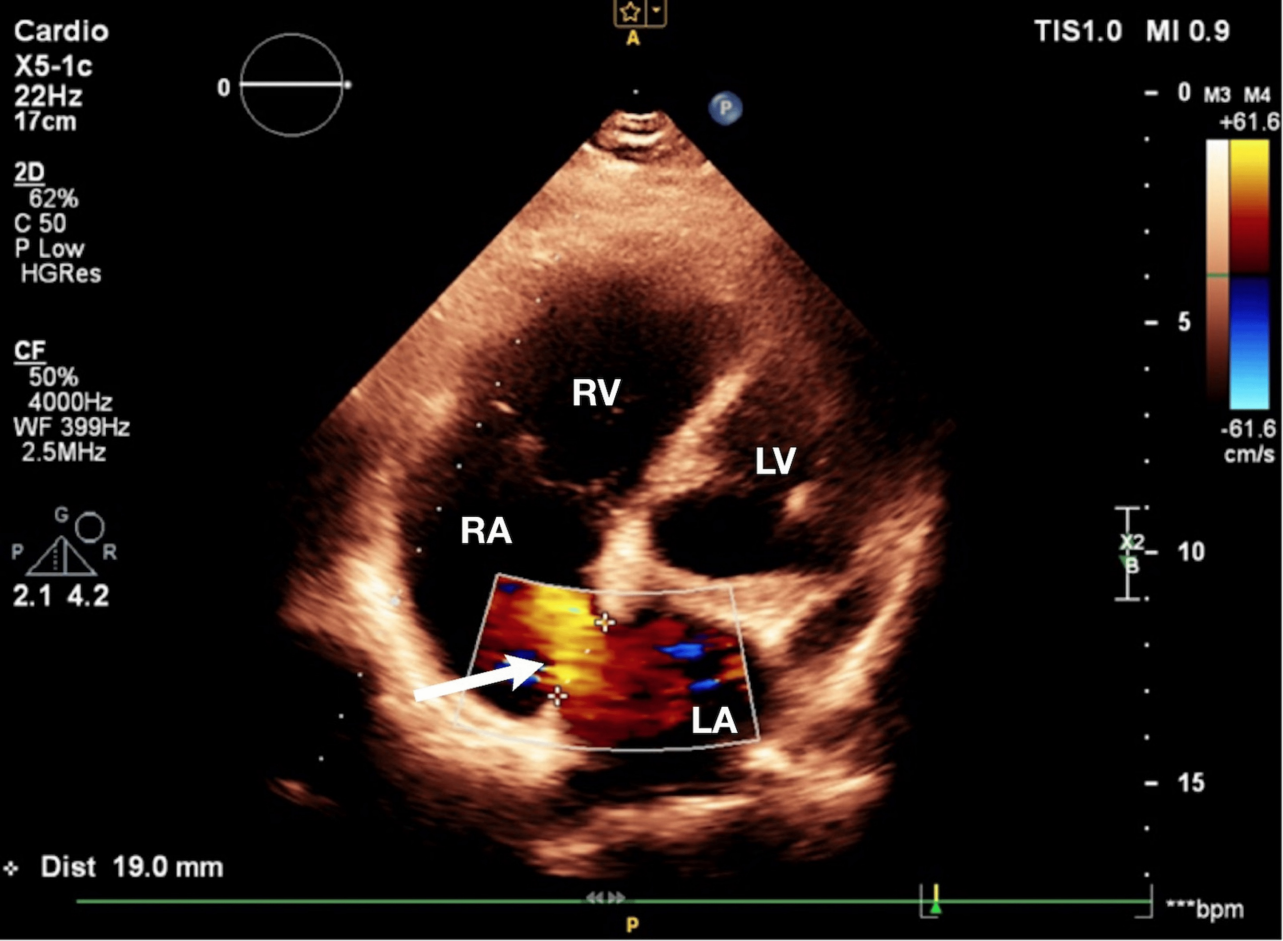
Transthoracic echocardiography with color Doppler demonstrating a secundum atrial septal defect (ASD II) with left-to-right shunting (apical four-chamber view). RV: right ventricle; RA: right atrium; LV: left ventricle; LA: left atrium
